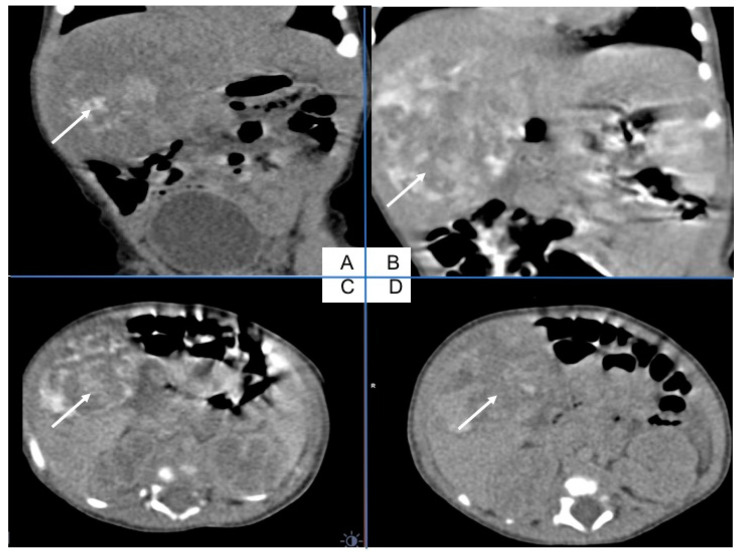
Abdominal CT with liver tumor in the right lobe. An abdominal nonenhanced (A) and enhanced arterial phase (B) coronal view, arterial phase (C) and portal phase (D) axial view indicate on the hepatic mass with bleeding (A), and central necrosis. After gadolinium contrast injection, there is poor peripheral enhancement in the arterioportal phase (C) and in the late portal venous phase CT image shows poor hypodense lesions (D).
